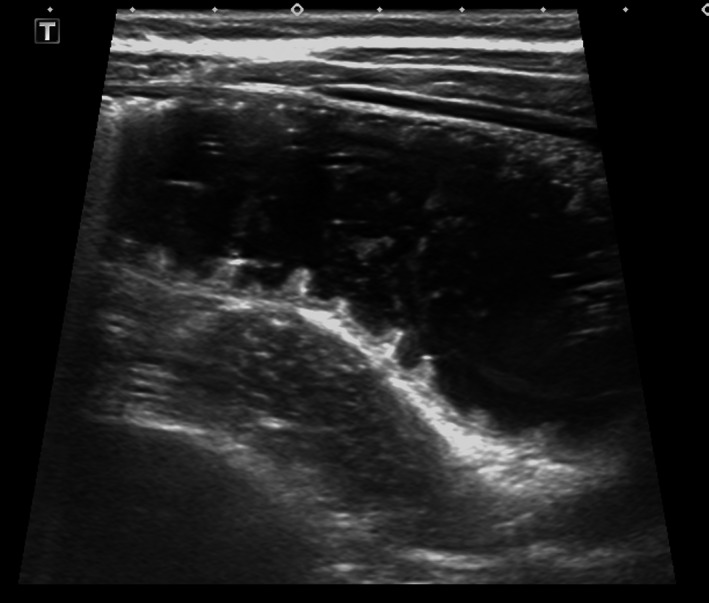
Ultrasound demonstrating distended and obstructed loop of proximal small bowel filled with heterogenous fecalized material, in keeping with acute obstruction
